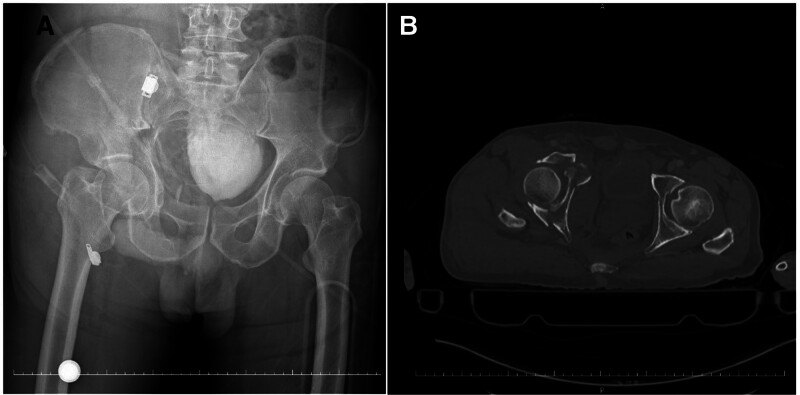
(A) Preoperative anteroposterior pelvic X-ray image. (B) Preoperative axial computed tomography image.
